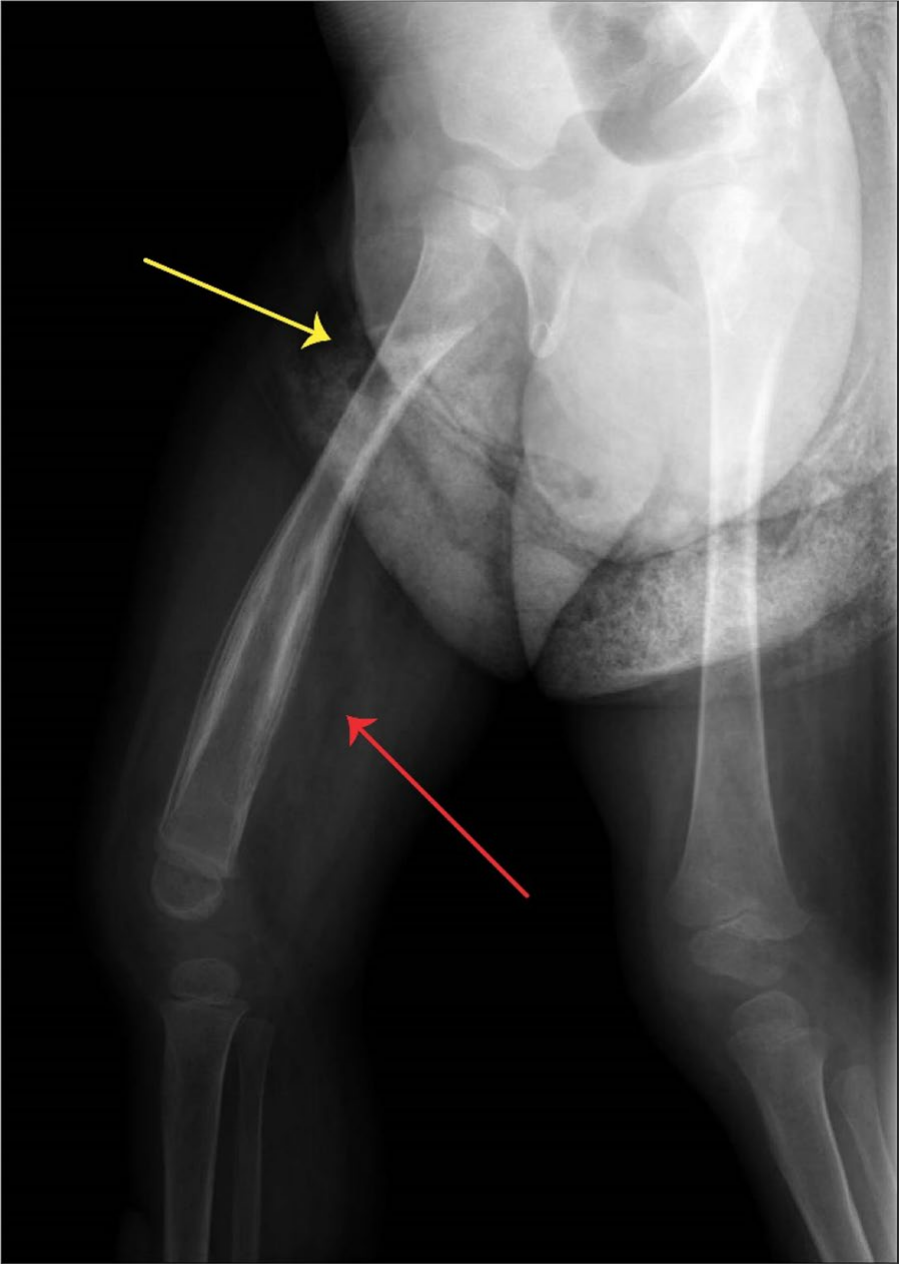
The X-ray of the patient shows signs of periosteal reaction in the diaphysis (red arrow) and a lucent area with sclerotic borders in the metaphysis of right femur (yellow arrow)
